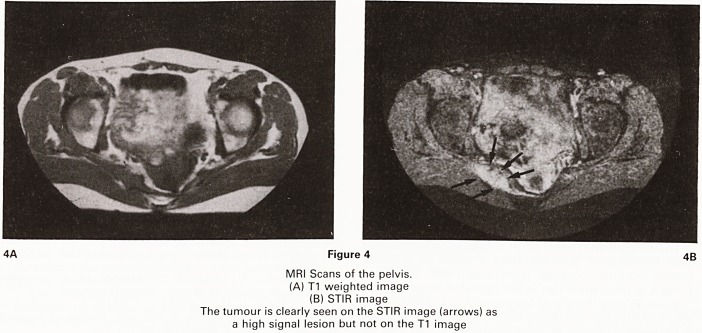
# The Detection of Recurrent and Metastatic Malignant Disease in the Pelvis Using MRI
